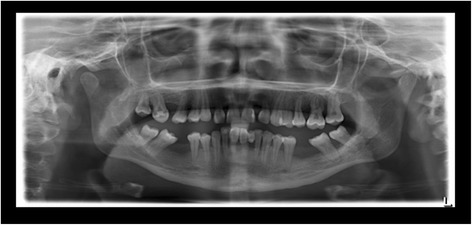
Panoramic radiograph at the age of 19 years: 3.5 years following full mouth supra- and subgingival debridement in combination with an adjunctive antibiotic therapy and supportive periodontal therapy every 3 months.
